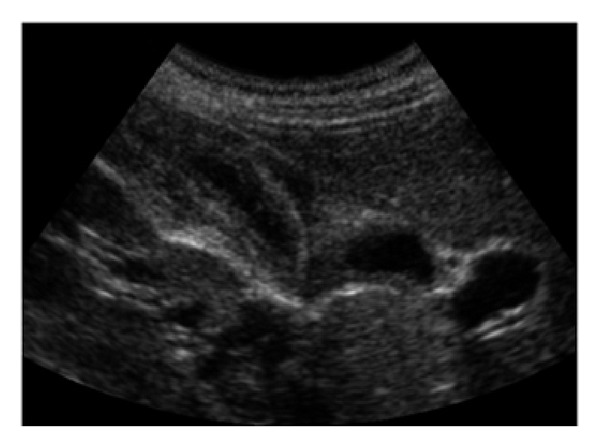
Ultrasound image. Thickening of the gallbladder wall. Minimal pericholecystic liquid is observed.
